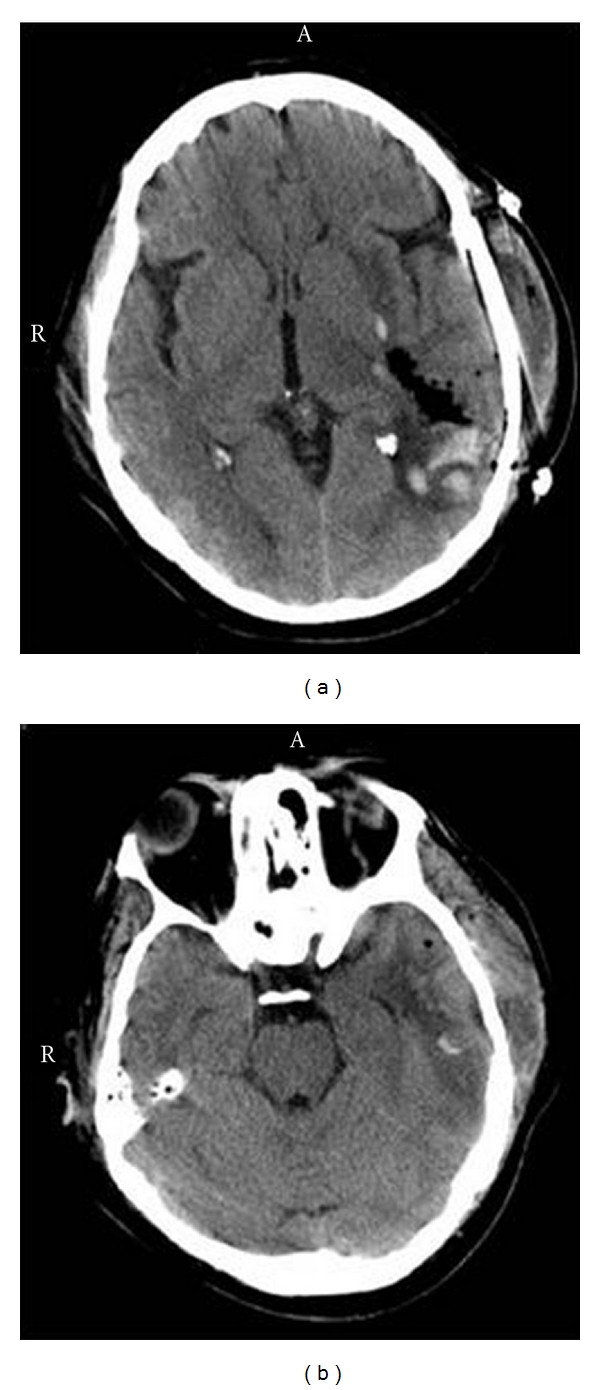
Participant no. 1 with a left temporoparietal lesion. The CT image in (a) depicts an axial scan through the maximum width of the lesion in the left angular gyrus. The dark area within the confines of the left hemisphere shows surgical evacuation of infarcted tissue within the left temporoparietal cortex. The CT image in (b) depicts the maximal width of the lesion in the left inferior temporal lobe. According to neuroradiological convention left side of the image is the right hemisphere and the right side of the image is the left hemisphere.
